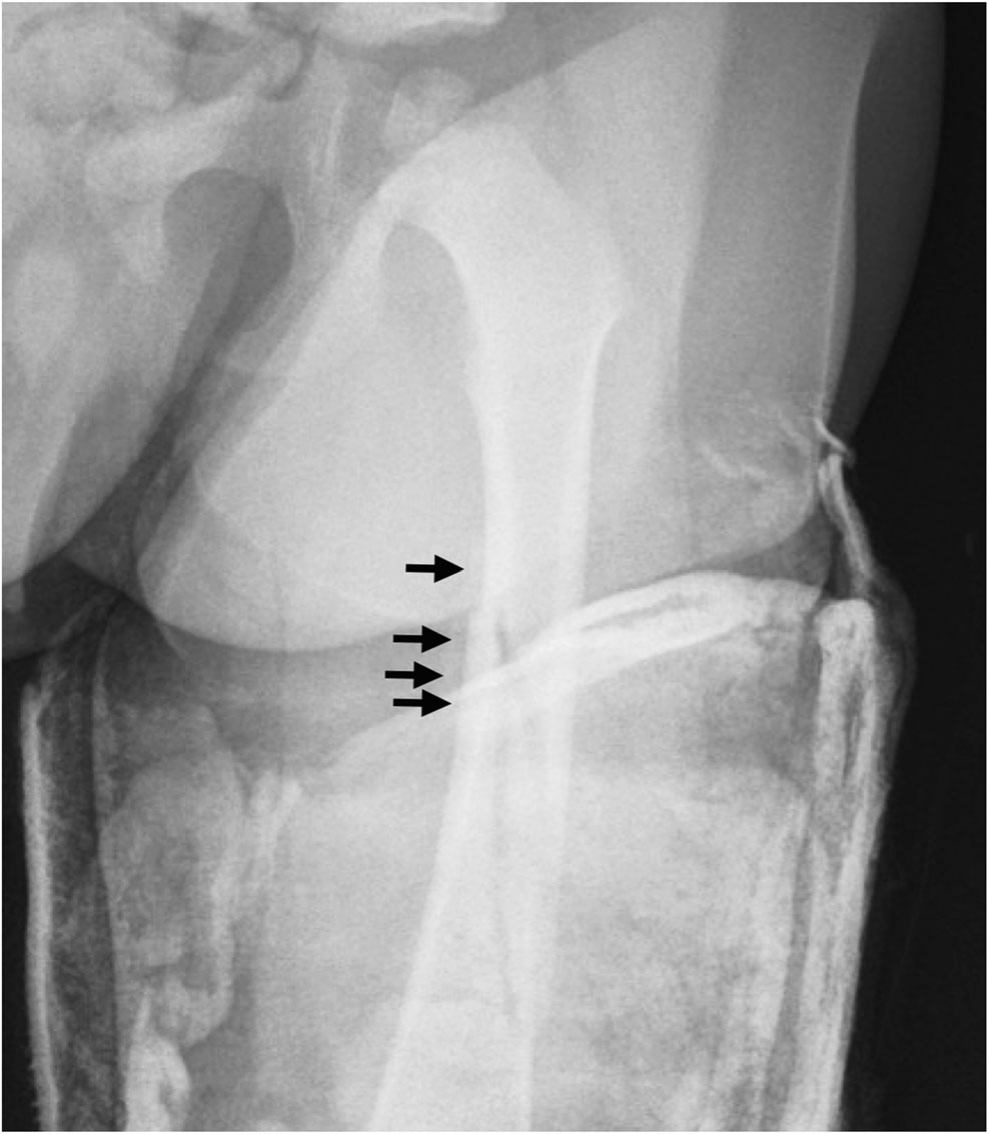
An anteroposterior radiograph of the left femur in a 22-month-old boy with the leg in a cast on the date of injury. The superior and medial edge of the cast (*arrows*) may easily be mistaken for subperiosteal new bone formation
